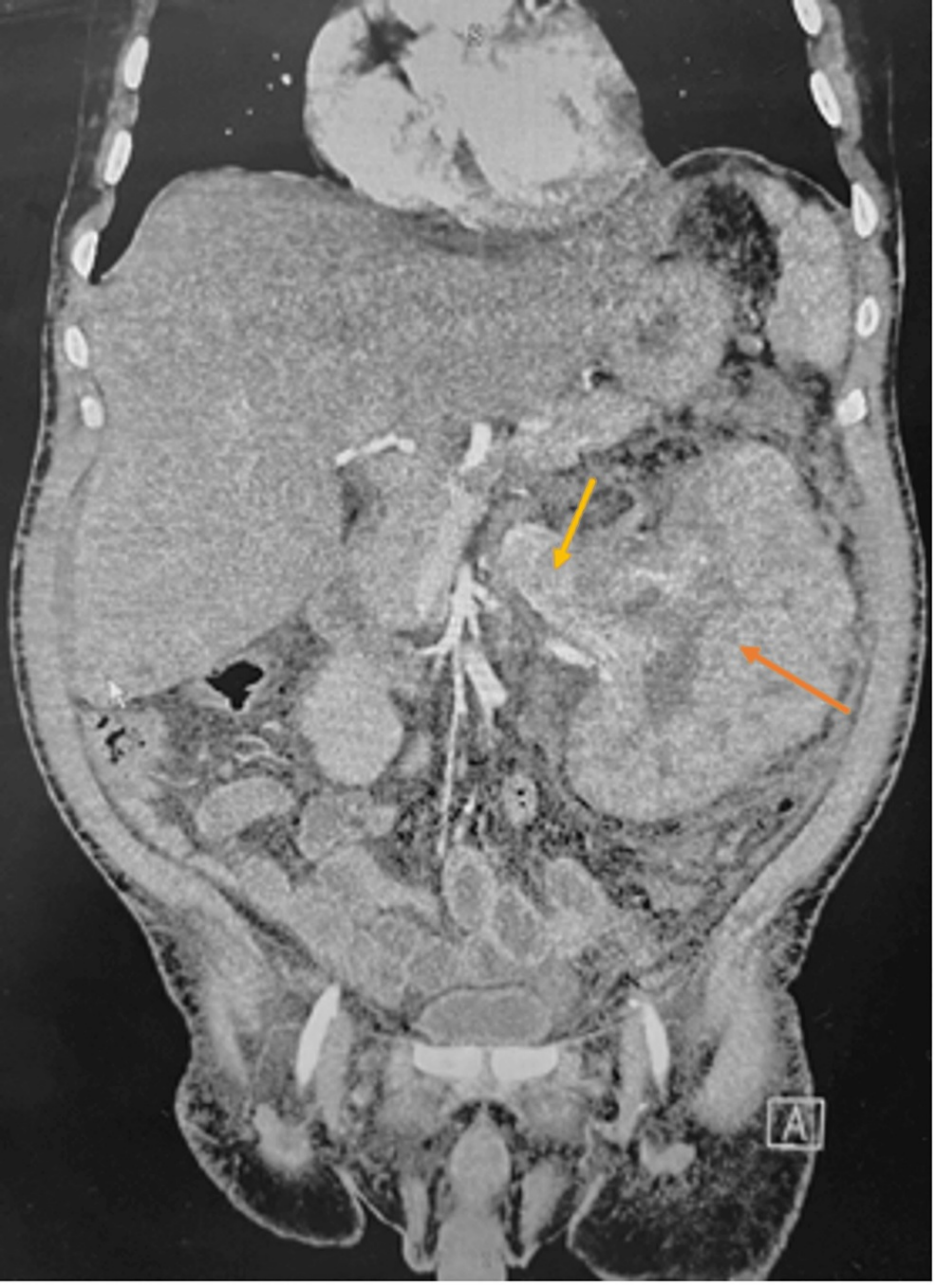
CTA of the abdomen and pelvis. CTA of the abdomen and pelvis demonstrating a large left renal mass (orange arrow) with the entire kidney in appearance and an enlarged left renal vein (yellow arrow). CTA, computed tomography angiogram
